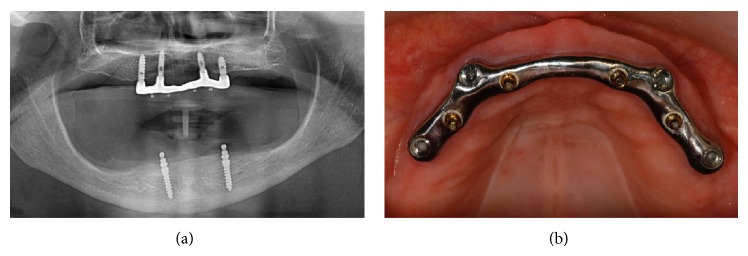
The maxillary overdenture (OD) at the 3-year follow-up: panoramic control radiograph (a); clinical view of the bar (b).
